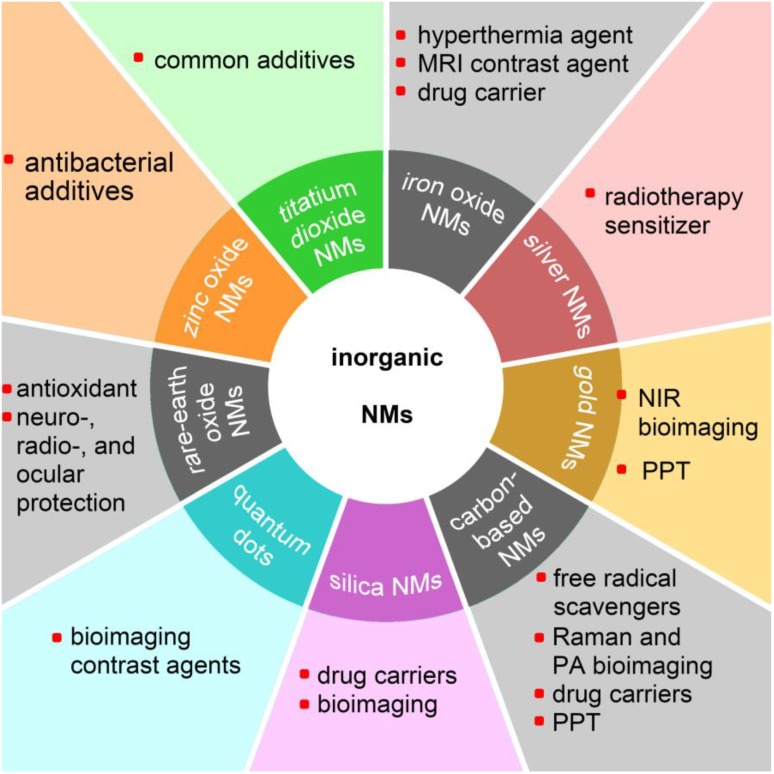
Potential applications of various inorganic nanomaterials (NMs) in the biomedical field. MRI: magnetic resonance imaging; NIR: near-infrared; PPT: photothermal therapy; PA: photoacoustic.
